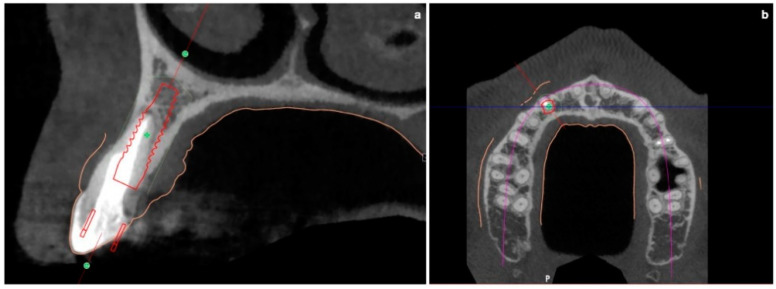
(a,b) Pre-surgical CBCT evaluation in order to perform guided implant insertion.
